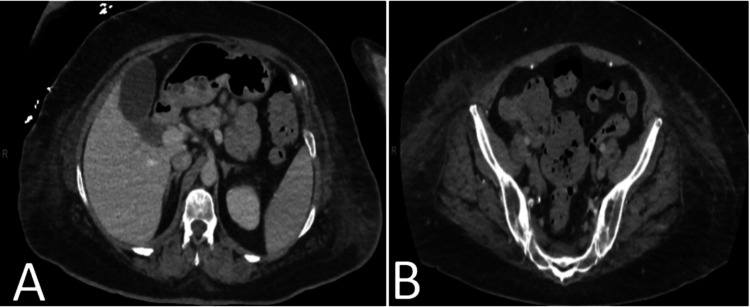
CT scan of the abdomen (A) and pelvis (B) showing no ascites CT: computed tomography
